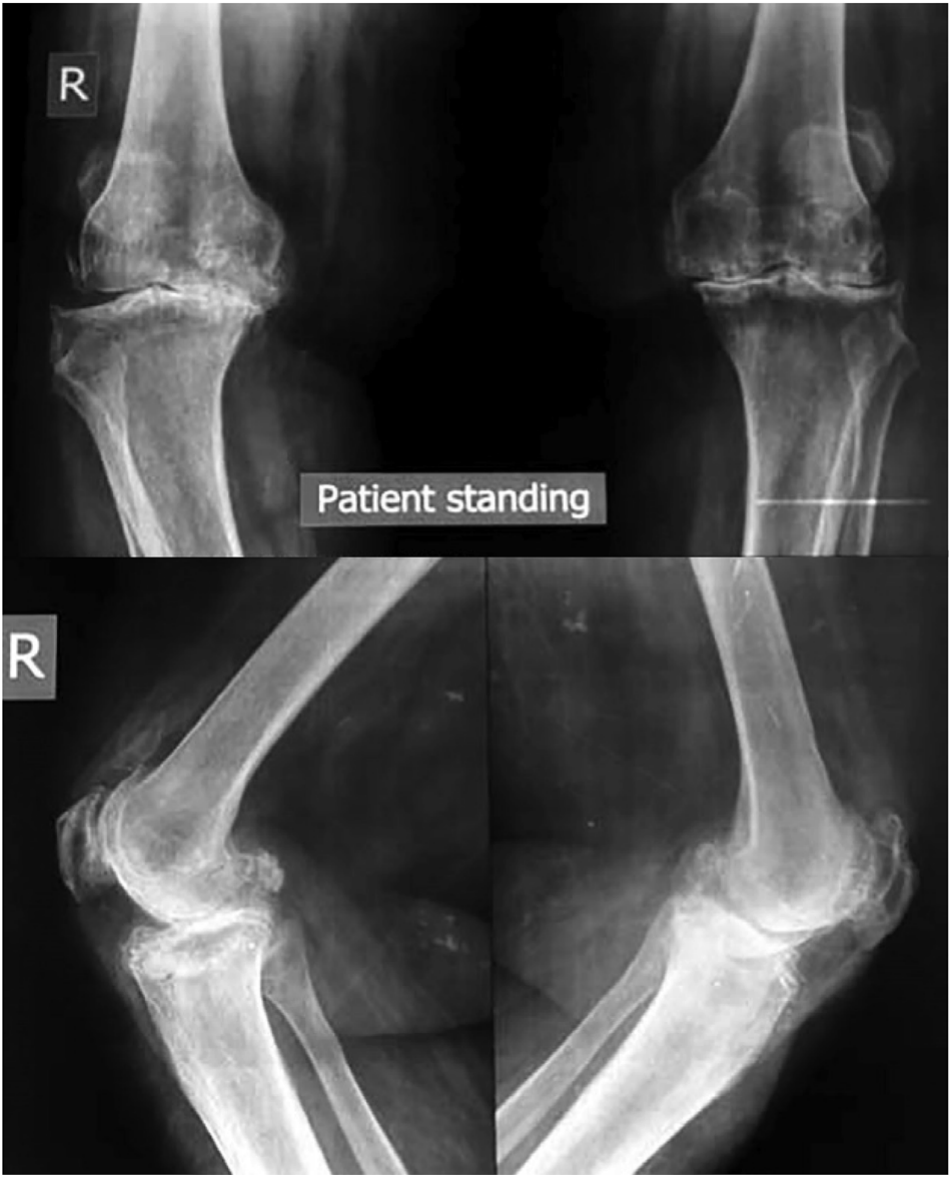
60 years old female, bilateral knee pain and difficulty walking. Preoperative x-rays showing advanced tricompartmental osteoarthritis and varus deformity.
